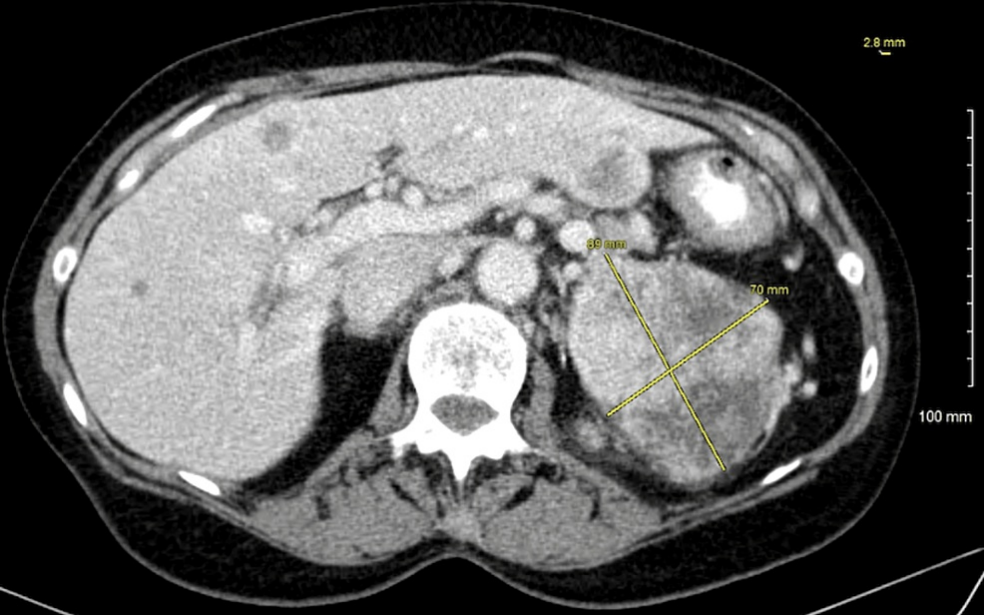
CT scan of the abdomen showing large heterogeneous soft tissue mass in the left renal bed measuring 8.9 × 7 cm. CT: computed tomography
